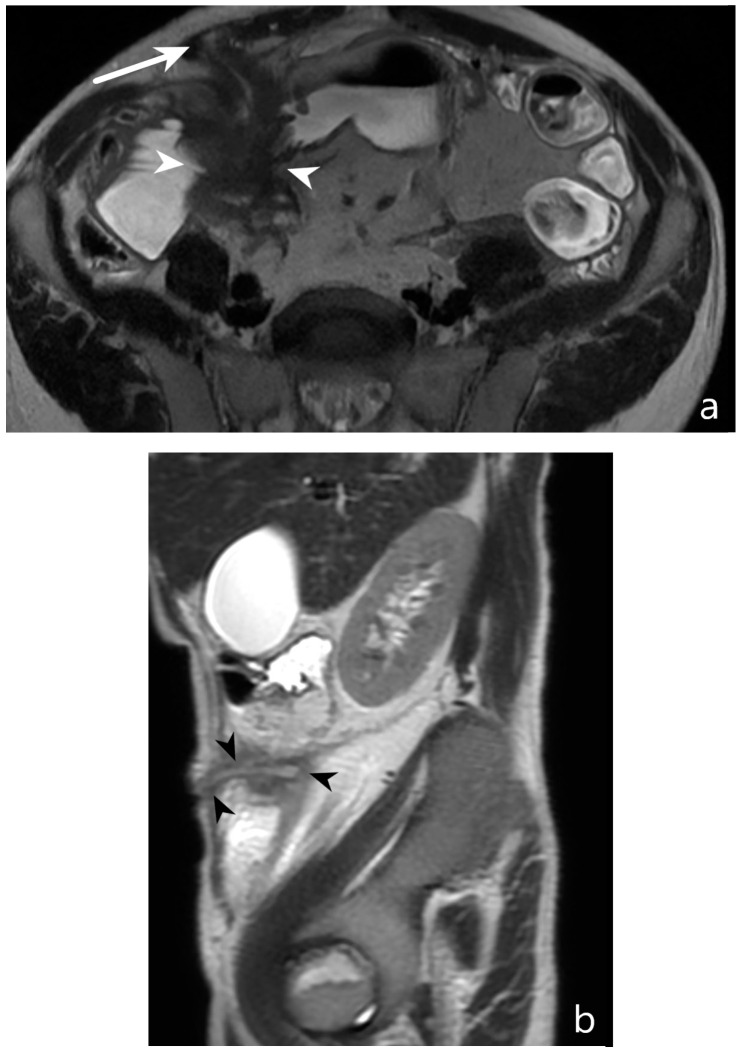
Entero-enteric fistula and entero-cutaneous fistula: MR-E after administration of polyethylene glycol solution. T2-weighted axial image (a) shows pathological loops with entero-enteric fistulas (white arrowheads). Further fistula with hyperintense T2 signal can be observed between the pathological loop and the anterior abdominal wall (arrow). T2-weighted sagittal image (b) shows the fistula between the pathological loop and the anterior abdominal wall; hyperintensity of signal in the lumen of fistula is present (black arrowheads). Original figures by LMM and LL.
